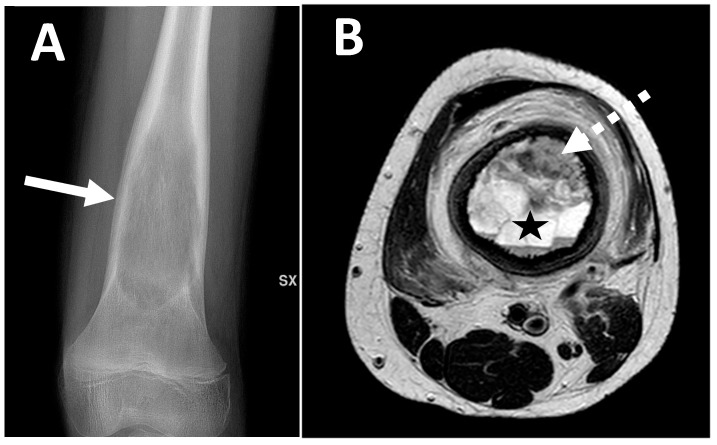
Conventional radiography of the left knee ((A) anteroposterior view) of a 13-year-old male with telangiectatic osteosarcoma of the distal femur metaphysis. A central expansile lytic lesion with limited multilayered periosteal reaction and sharply ill-defined margins can be observed (arrow). MRI ((B) axial T2-WI) shows multiple fluid–fluid levels with septa (star) and a concomitant solid area within the anterior aspect of the lesion (dotted arrow).
